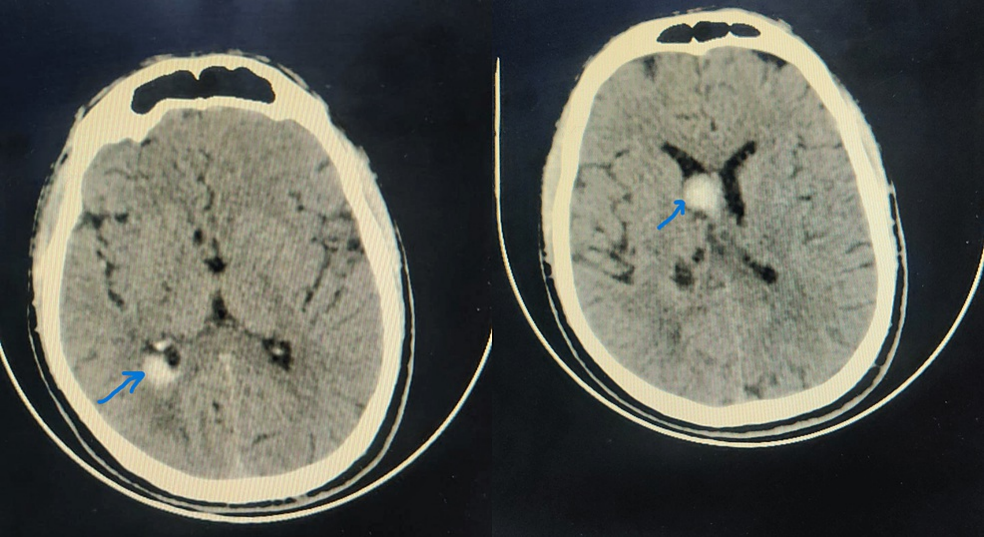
NCCT head showing bleeding in the periventricular white matter around the right lateral ventricle with extension to the lateral ventricle NCCT: non-contrast computed tomography
